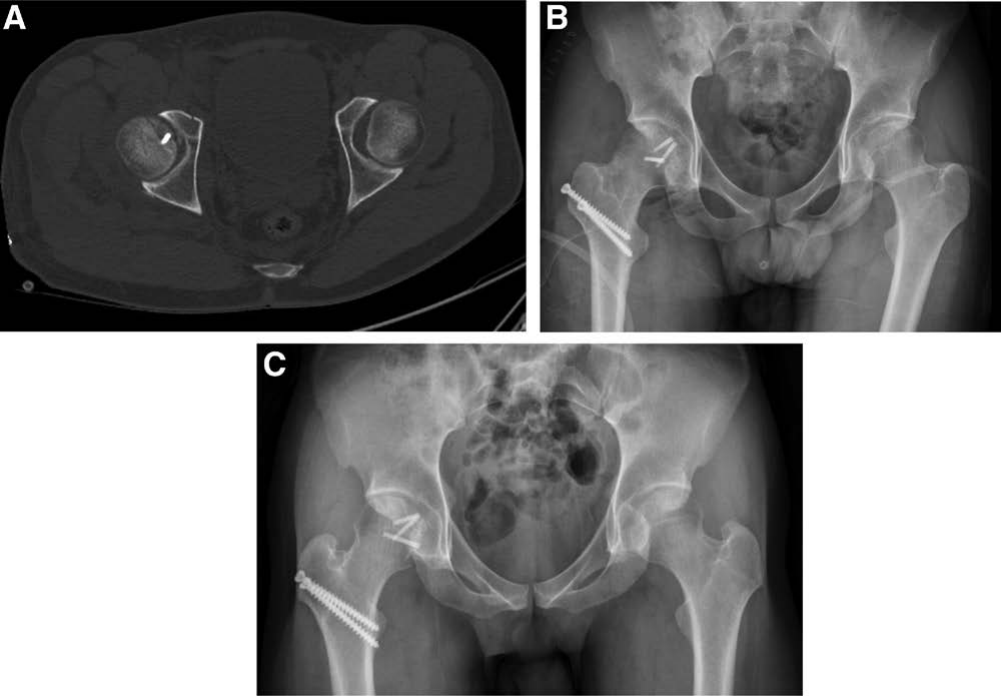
(A, B): Immediate post-operative computed tomography and Hip Anteroposterior x-ray showing a congruent femoral head. (C): Anteroposterior hip x-ray at 5 years postoperatively shows no evidence of collapse or arthritic change in the femoral head.
